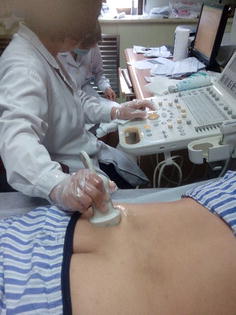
The vascular malformation of the superior gluteal artery were checked by routine preoperative hip vascular ultrasound
